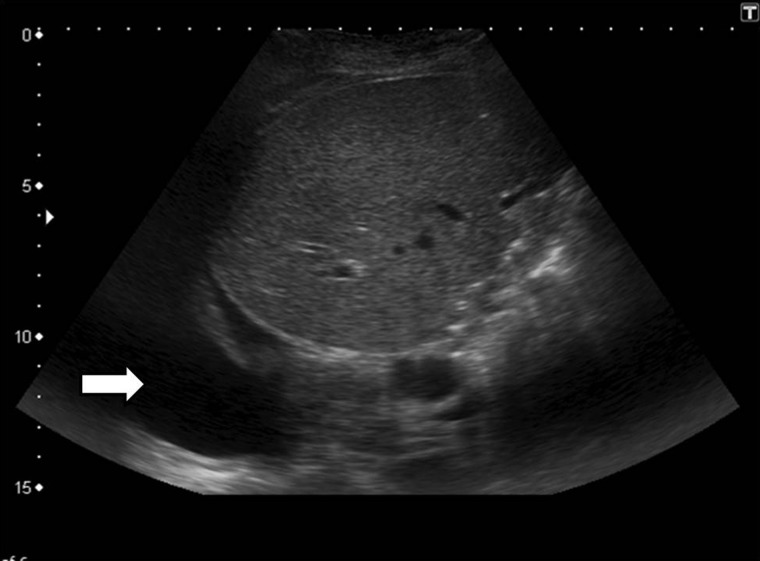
Pleural effusion. Ultrasound image showing right pleural effusion (arrow) with passive atelectasis.
